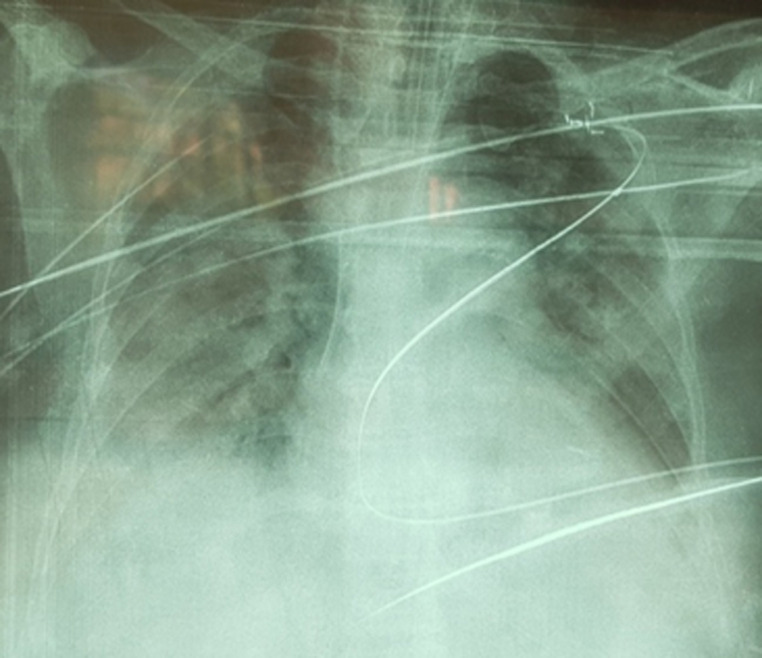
post-intubation chest X-ray
